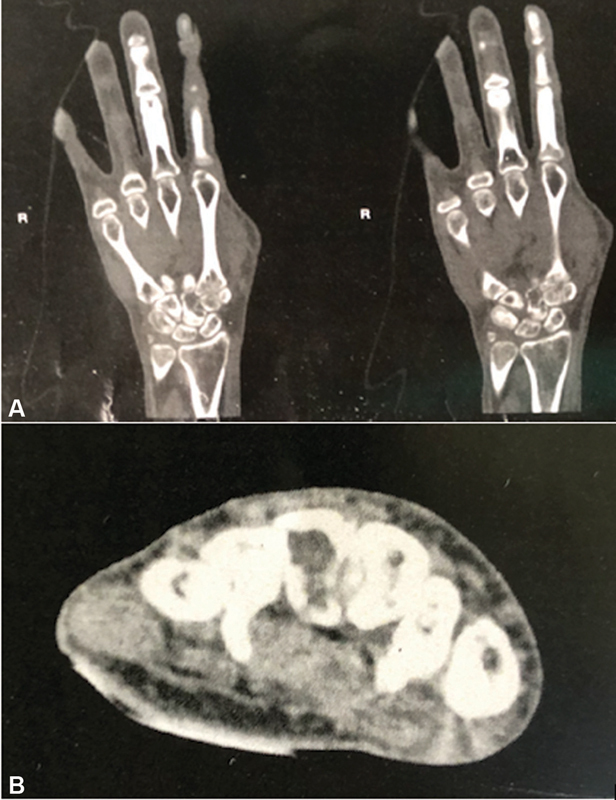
Computed tomography of the right hand. Coronal (
